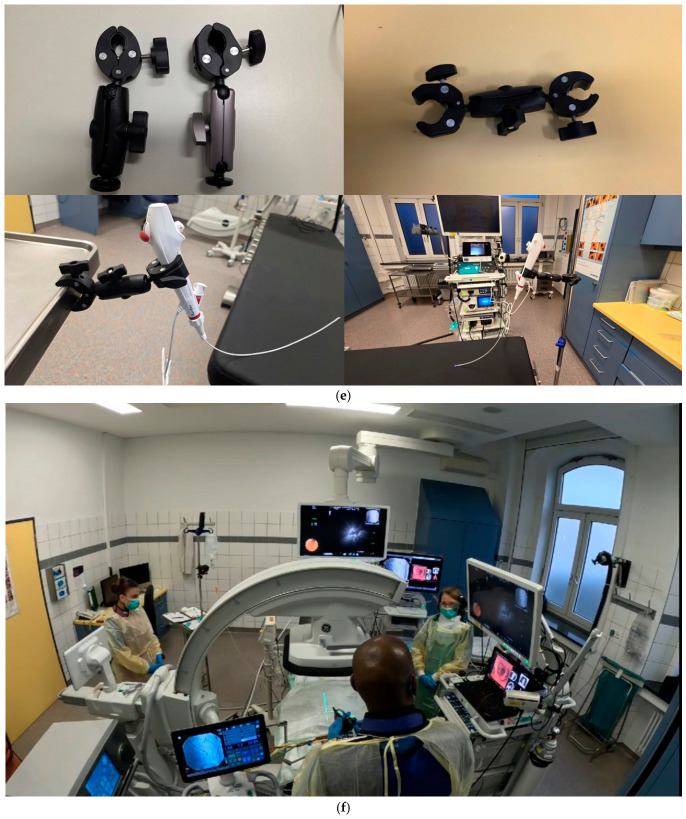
(a,b): Standard flexible bronchoscope holder/fixation systems. (c): Tracheoscope mounting system used as bronchoscope stabilization device. (d): Single-use bronchoscope taped to patient during CBCT spin. (e): Action camera mounting systems converted to bronchoscope holders to mount bronchoscope to stationary objects. (f): Room layout during CBCT interventions.
